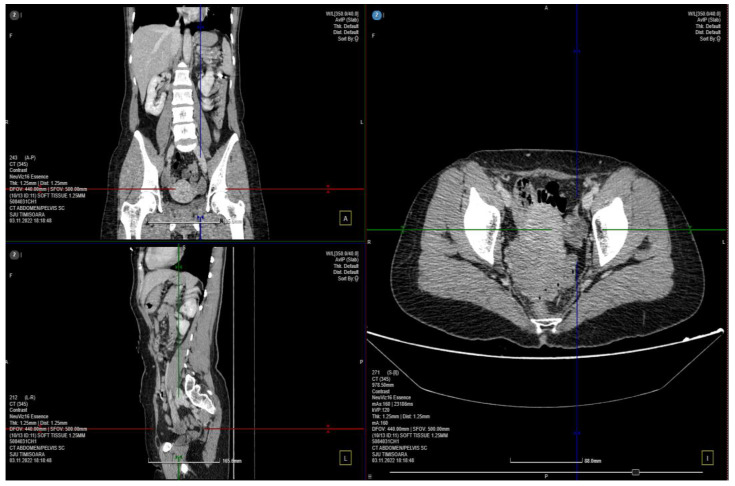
CT scan of the abdomen and pelvic 3 different plans: the coronal, sagittal and axial planes.
